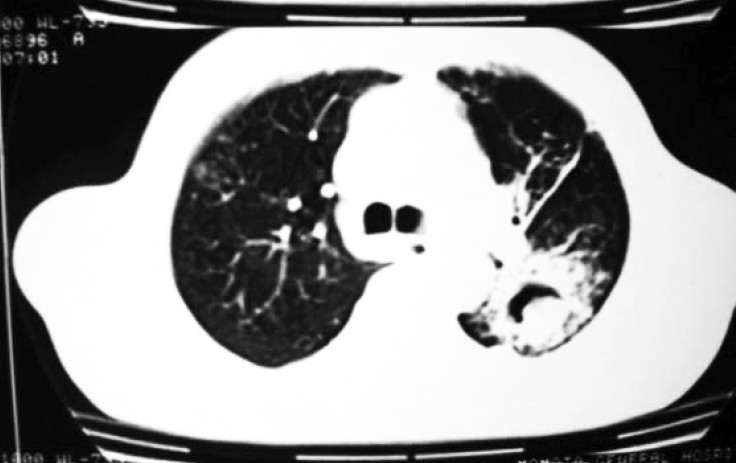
CT thorax showing mycetoma with air crescent in left
